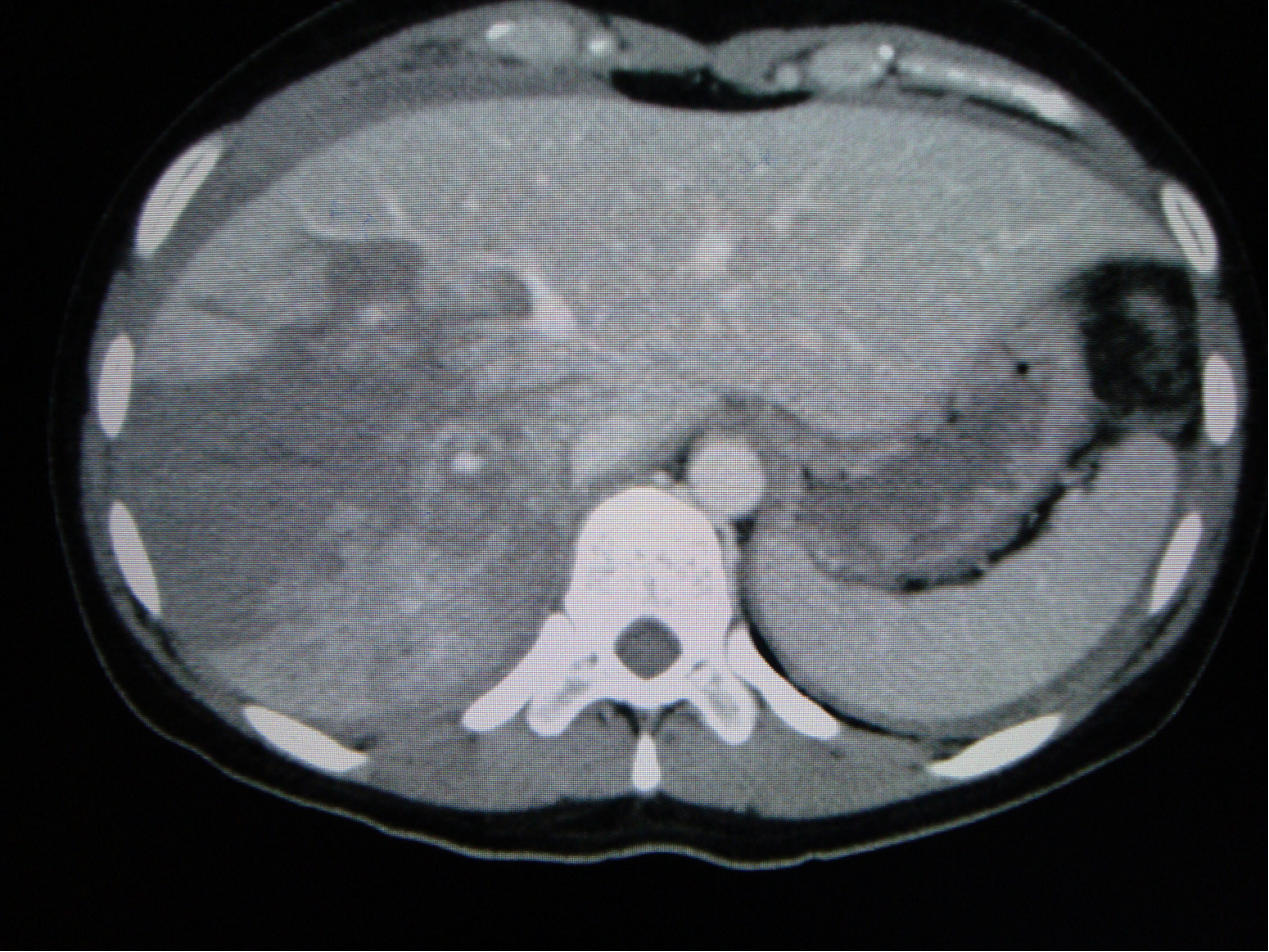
Bicycle crash; multisclice CT showing the presence of abdominal free fluid, with intraparenchymal hematoma in the right lobe (grade IV hepatic injury), no blush of contrast in the arterial phase.
